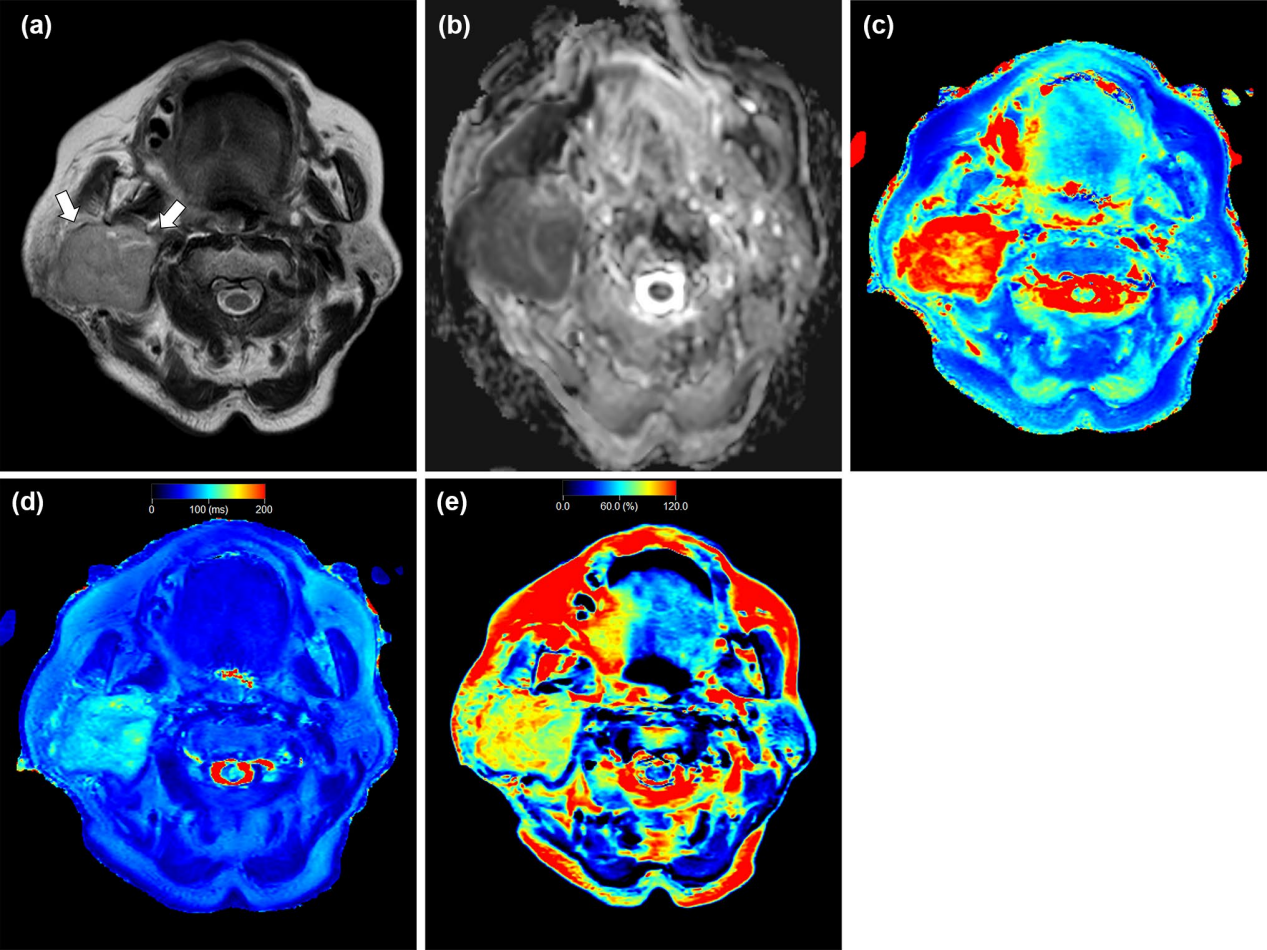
A 72-year-old female with carcinoma ex pleomorphic adenoma. T2-weighted image (a) shows a lesion with lower signal intensity (arrows) relative to parenchyma in the adjacent right parotid gland. Apparent diffusion coefficient (ADC) (b), T1 (c), T2 (d), and PD (e) maps show the lesion, which has a mean ADC value of 0.77 × 10^−3^ mm^2^/s, mean T1 value of 1869 ms, mean T2 value of 92 ms, and mean PD value of 86%
